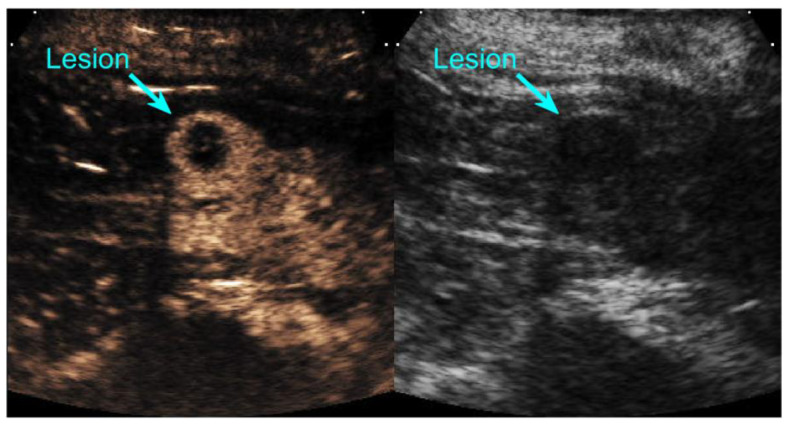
Dual split-screen ultrasound image of a kidney lesion in contrast mode (left side) and B-mode (right side). The lesion is labeled with a cyan blue arrow.
